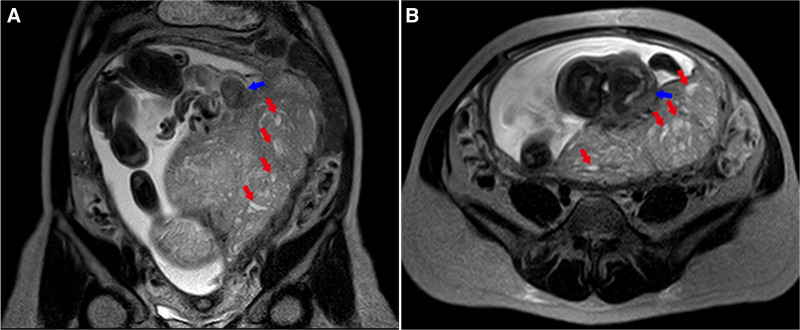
MRI of PMD at 32 wk and 2 d of gestation. (A) T2-weighted sagittal MRI image of PMD. (B) T2-weighted transverse MRI image of PMD. (Red arrows: highlight hypoechoic areas within the placental parenchyma. Blue arrows: vascular tumors at the base of the umbilical cord on the fetal side of the placenta.) MRI = magnetic resonance imaging, PMD = placental mesenchymal mysplasia.
